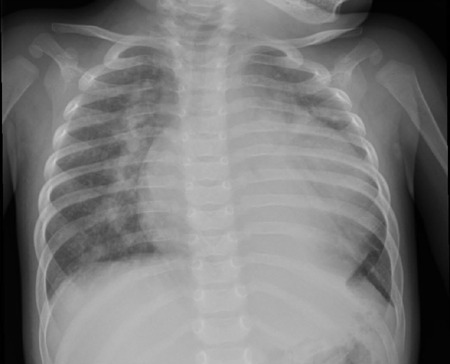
Chest X-ray demonstrating significant cardiomegaly and attenuated
